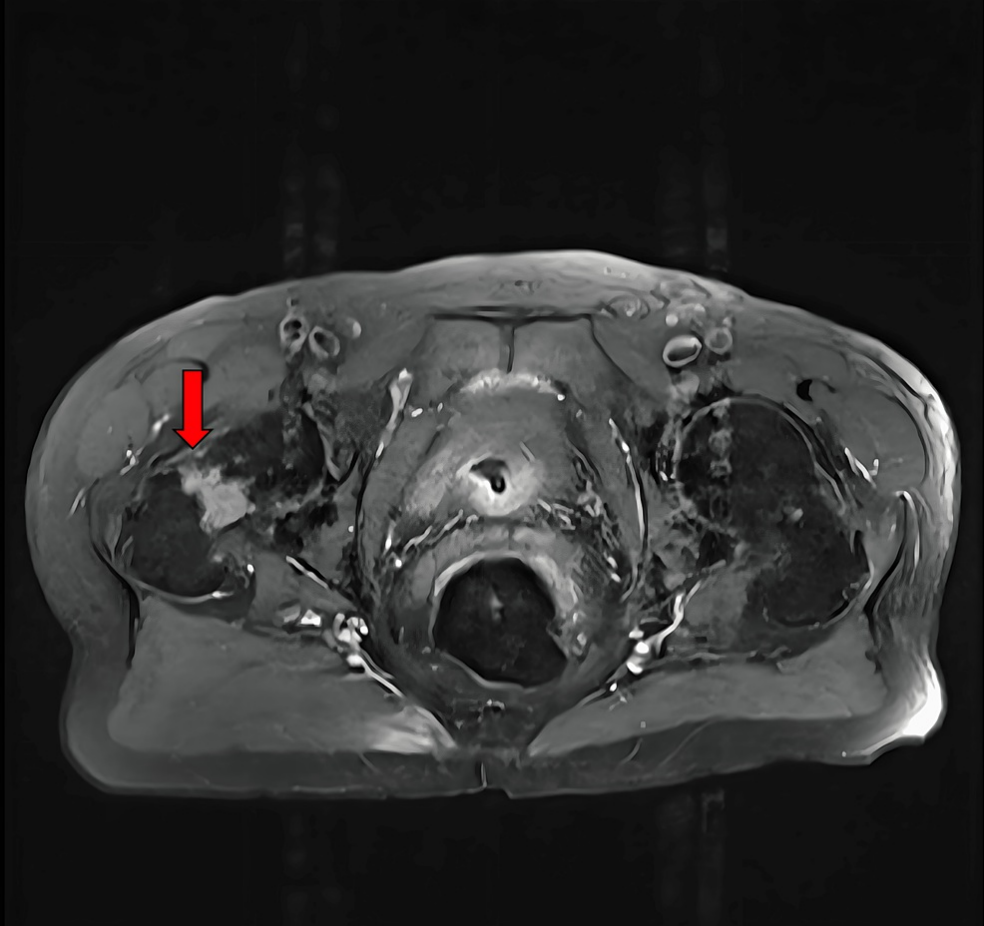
T1-weighted pelvic MRI with contrast, axial view An enhancing marrow-based lesion involving the right femoral neck is present with extension into the cortical bone (arrow). The location of this lesion indicates a high risk in this patient for a pathologic fracture with unassisted ambulation.
